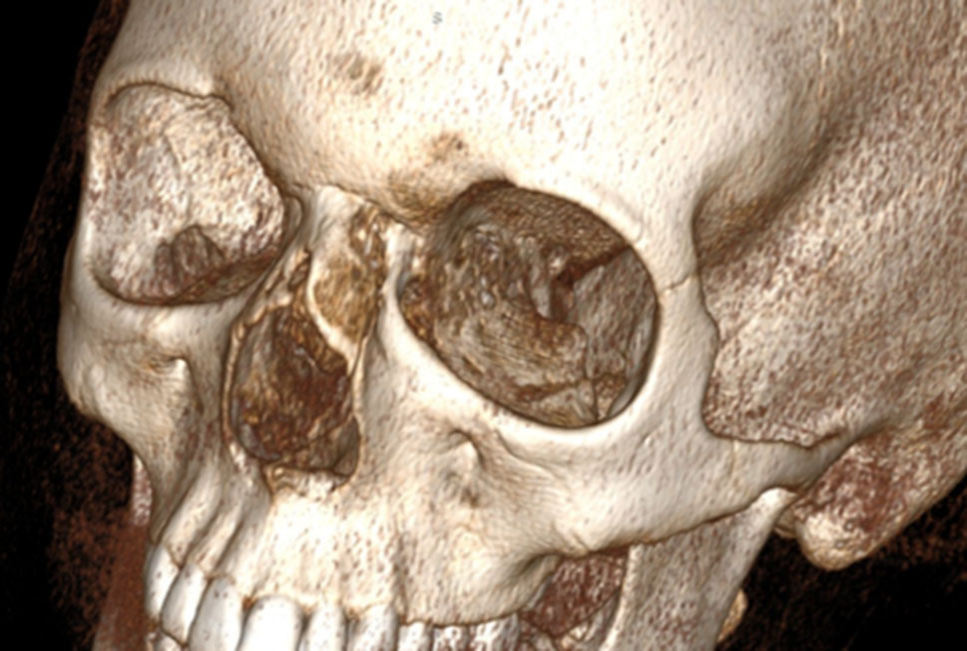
Deformation and atrophy of the nasal bones in 12-year-old girl with nasal dermoid—CT scan & 3D reconstruction
